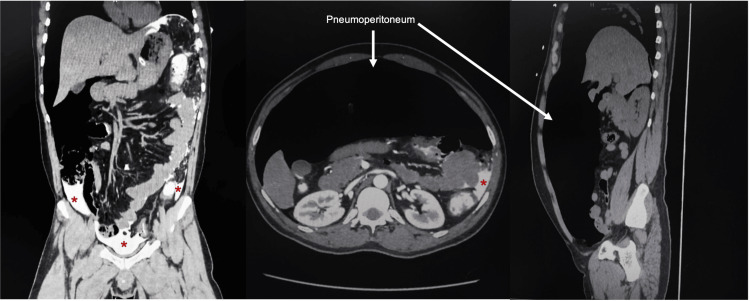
Abdomen CT with IV and rectal contrast Marked pneumoperitoneum in addition to rectal contrast extravasation at the rectosigmoid junction into the pelvis, paracolic gutter, and perisplenic region indicated by red asterisks.
